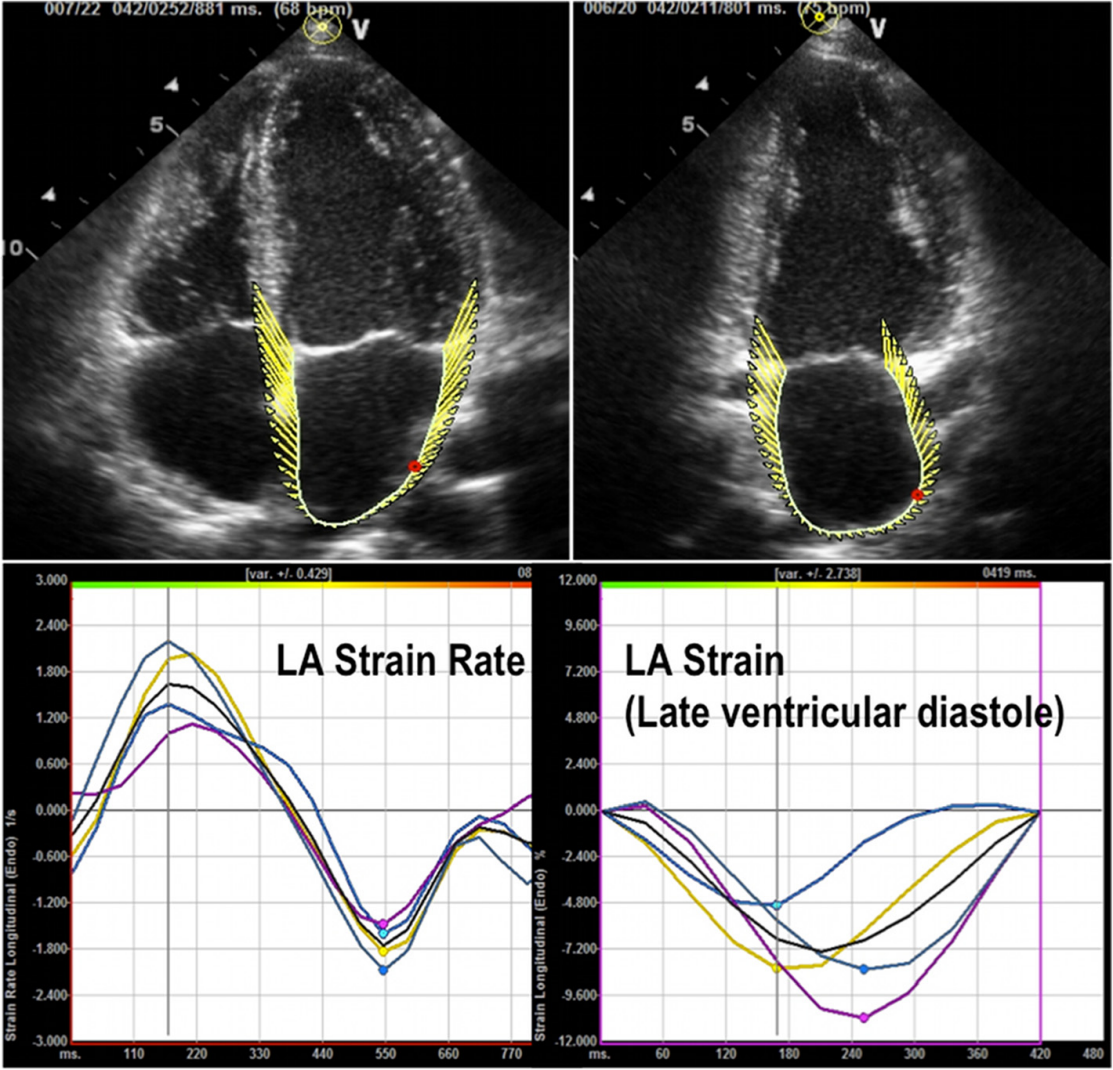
Velocity vector imaging for left atrial strain and strain rate in the apical 4-chamber *(left images) *and apical 2-chamber *(right images) *views. The right lower image is an example of LA strain during atrial systole and the left lower image is an example of LA strain rate curves. In the left lower image, the black line represents the global LA strain rate during LV systole, early and late diastole. The colored lines represent the strain rates of the two annular and mid segments of the LA. The right lower image shows the global and segmental LA strain during atrial systole (late ventricular diastole).
